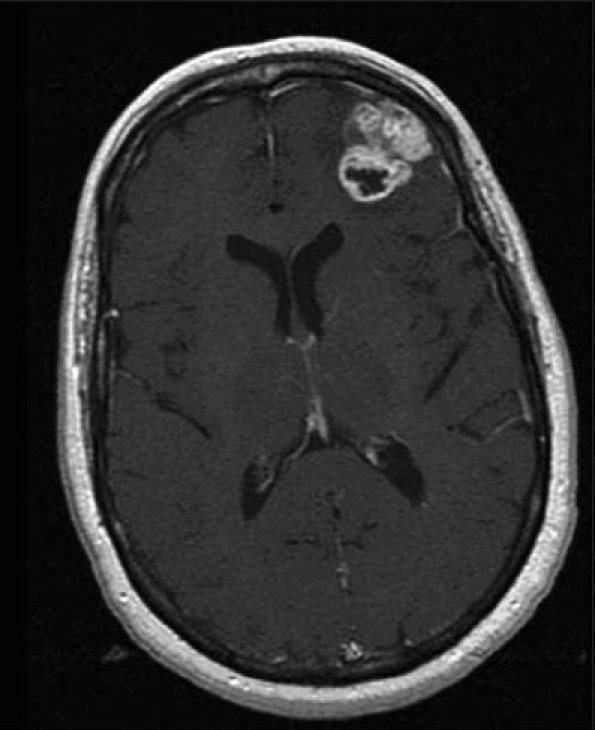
T1-weighted MR images with gadolinium. Nonhomogeneously enhancing left frontal tumor
